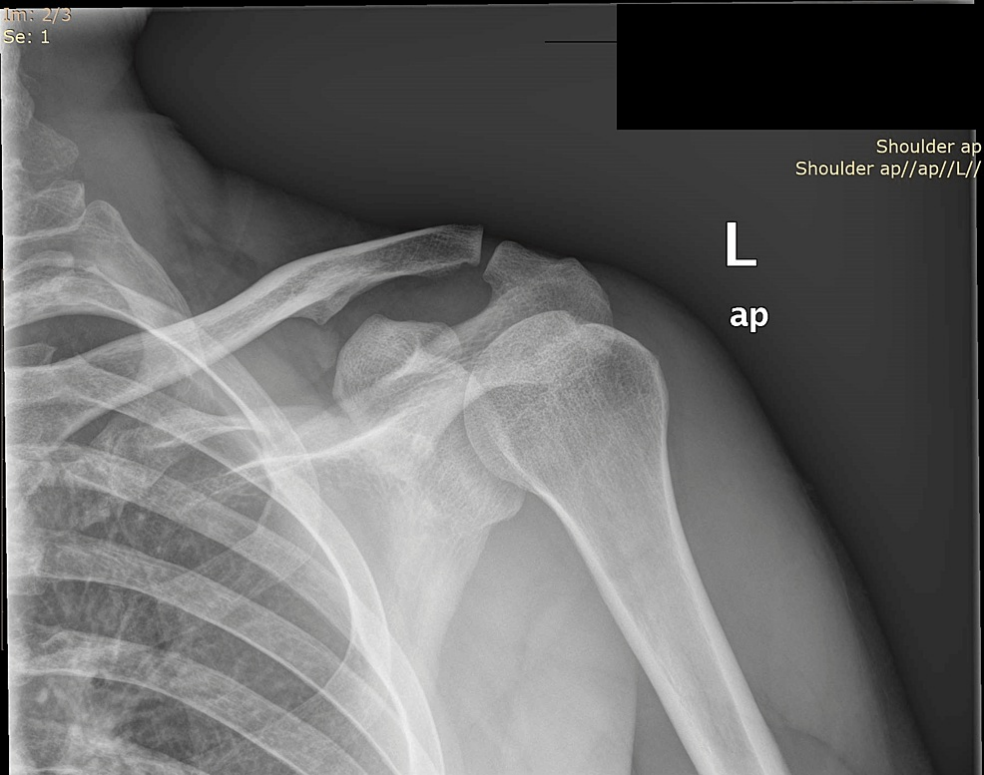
Anteroposterior X-ray of the left shoulder after close reduction by two-step technique maneuver
